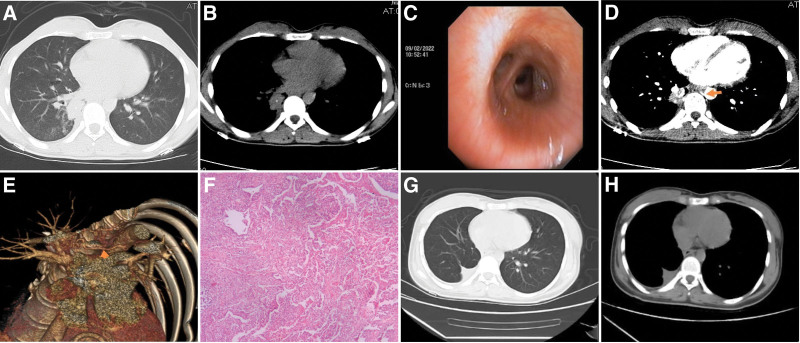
(A, B) The chest CT scan revealed a dense mass-like shadow in the basal segment of the right lower lobe of the lung near the paraspinal column, accompanied by foci of calcification. (C) Bronchoscopy identified congestion and the presence of yellow mucous sputum adhering to the opening of the right lower basal segment. The examination did not reveal any stenotic neoplasms or obstructions within the lumen. (D, E) Enhanced CT of the chest, along with a 3-dimensional reconstruction of the pulmonary vasculature, demonstrated that the arterial blood supply to the right lower basal segment of the lung originated from the thoracic aorta (arrows indicate the abnormal arteries supplying the blood). (F) The postoperative pathology of the right lower lung lesion showed neutrophilic granulocyte exudation in the alveolar lumen, bronchioles, and fine bronchial lumens. The bronchial lumens exhibited radially arranged hyphae, with the tips of the hyphae expanding in a pestle and mortar-like pattern. Some hyphae appeared wrapped up in a mass, consistent with actinomycosis infection. (G, H) A follow-up chest CT on January 17, 2024, revealed postoperative changes in the right lower lung field, with the condition showing stability.
